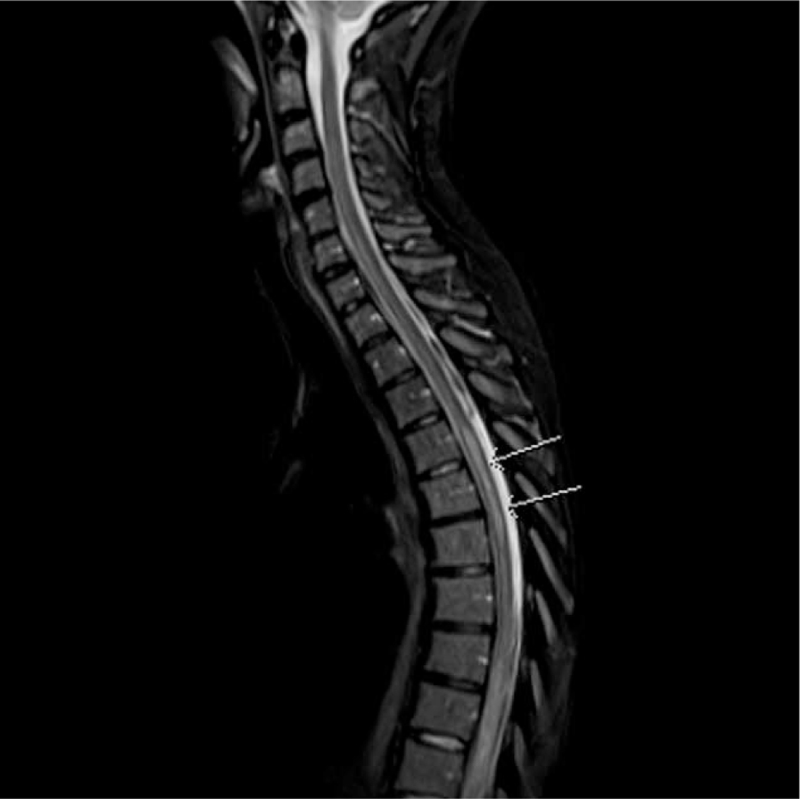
Sagittal STIR image of MRI spine demonstrated increase signal from midthoracic extending to the level of conus medullaris. MRI = magnetic resonance imaging.
